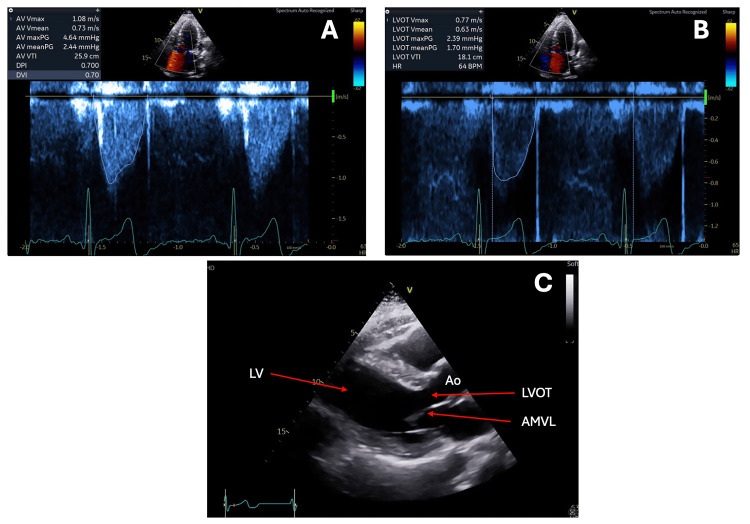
Transthoracic echocardiography (TTE) views obtained six months post-liver transplantation. (A) Parasternal long-axis (PLAX) view in late systole demonstrating absence of systolic anterior motion of the mitral valve. No mid-ventricular cavity obstruction is observed. (B) Pulse-wave Doppler interrogation at the left ventricular outflow tract (LVOT) level revealing normal flow velocities without evidence of obstruction. Continuous-wave Doppler assessment demonstrates normal gradients across the LVOT, mid-cavity, and aortic valve.
